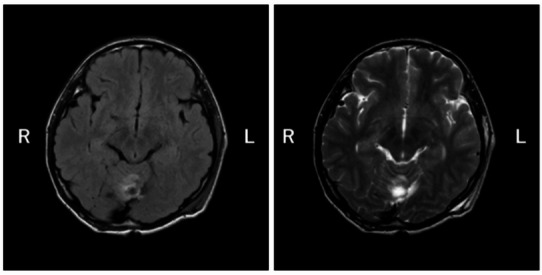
Postoperative T1- and T2-weighted MRI images. Postoperative T1- and T2-weighted brain MRI confirm the resection of the cerebellar vermis mass
